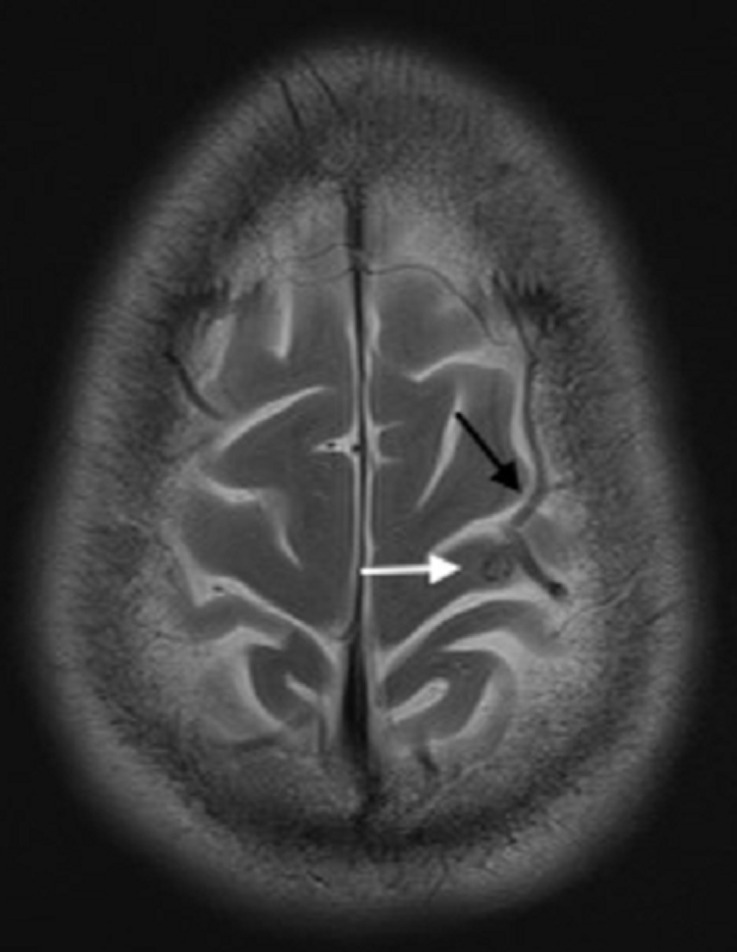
axial T2-weighted magnetic resonance imaging brain showing a non-thrombosed developmental venous anomaly in the left frontal region (black arrow) adjacent to a cystic lesion typical of vesicular-stage neurocysticercosis (white arrow)
